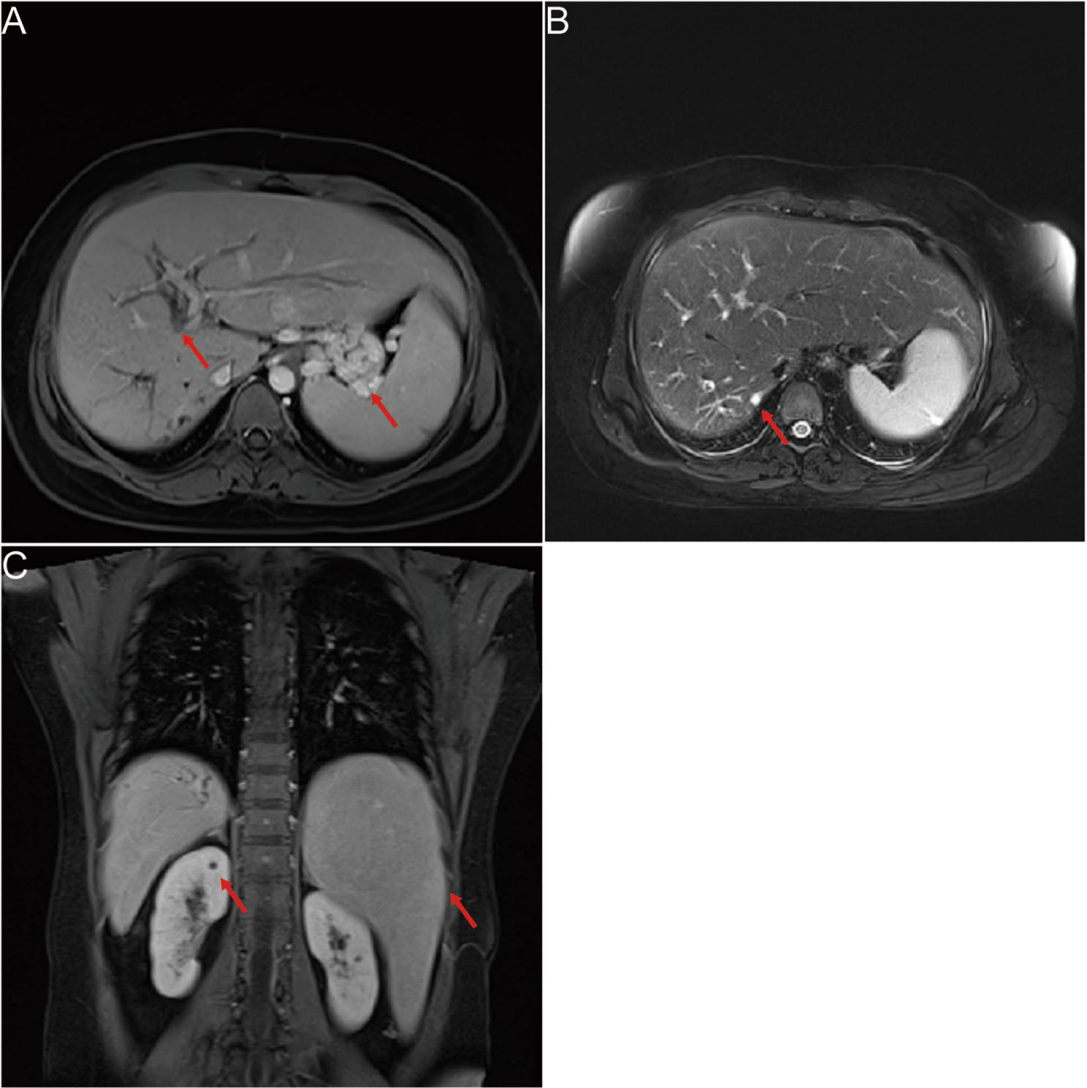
Imaging findings at discharge and follow-up results of the patient. (A) MRI imaging revealed intrahepatic bile duct dilatation and gastric fundic varices (arrow). (B) MRI imaging revealed cystic dilatation of the intrahepatic bile duct (arrow). (C) MRCP imaging showed the morphological characteristics of bile duct tree
